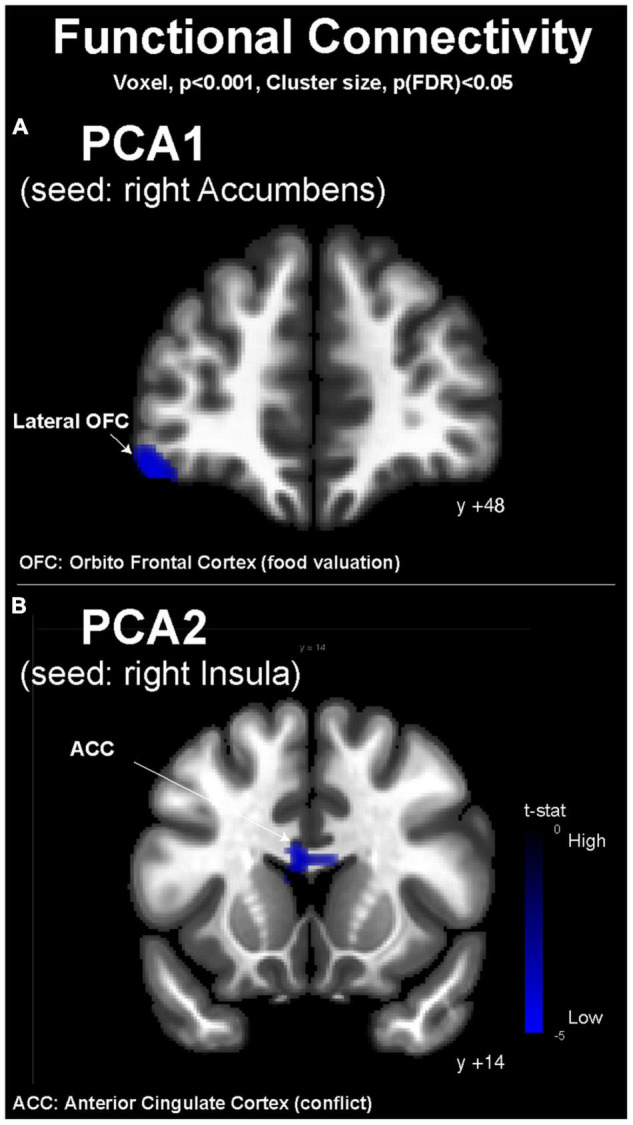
Resting-state functional magnetic resonance imaging functional connectivity maps (conn toolbox). (A) Depending on the PCA1 values with the right accumbens as seed and (B) depending on PCA2 values with the right insula as seed. MNI coordinates are indicated for each coronal slice. OFC, orbitofrontal cortex; ACC, anterior cingulate cortex.
